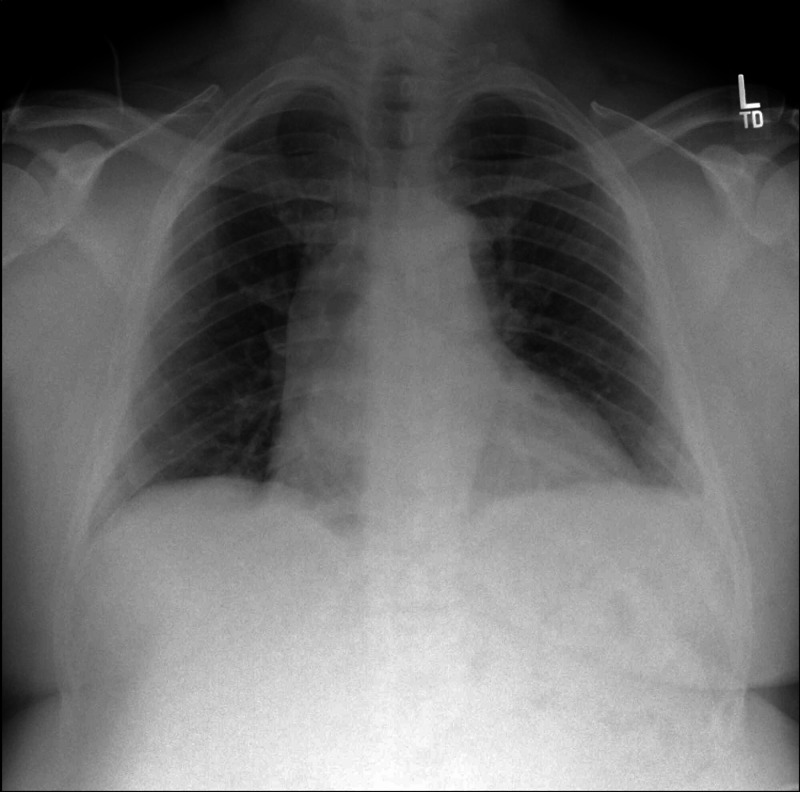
Chest X-ray showed mild cardiomegaly
